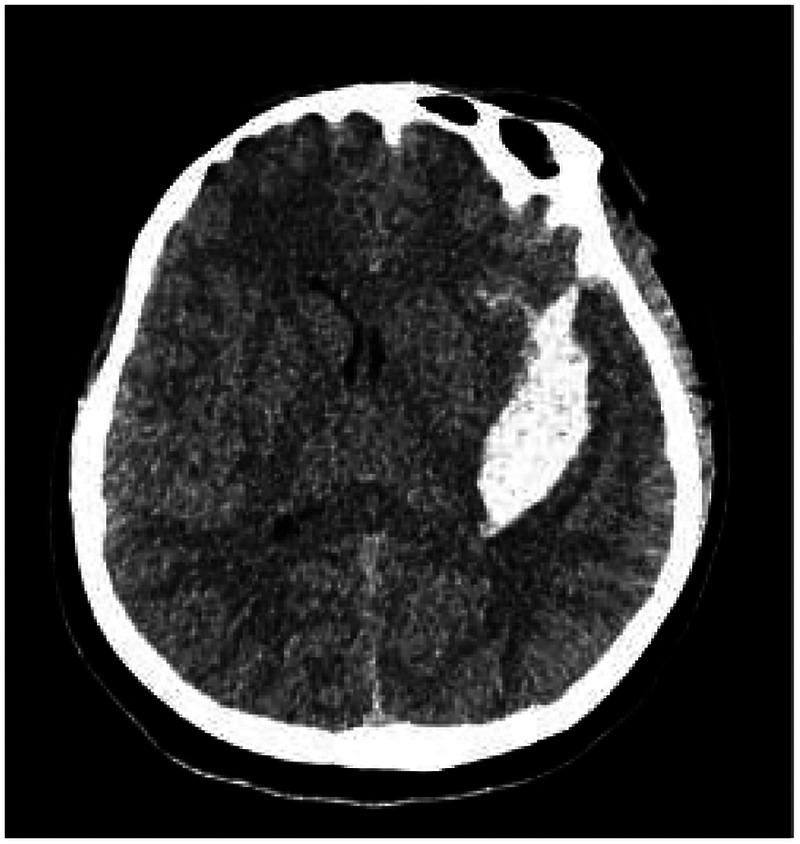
CT demonstrates a hyperdense lesion in the left temporal-insular region with surrounding edema, consistent with intracerebral hemorrhage causing mild midline shift.
